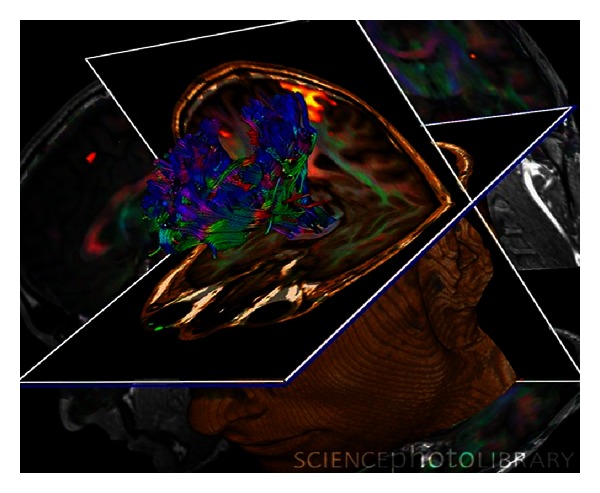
Brain tumour. Coloured 3D diffusion tensor imaging (DTI) and magnetic resonance imaging (MRI) scans of the brain of a 29-year-old with a low-grade glioma in the left frontal lobe. A DTI scan shows the bundles of white matter nerve fibers and is being used here for presurgical planning. The fibers transmit nerve signals between brain regions and between the brain and the spinal cord. A glioma arises from glial cells; nervous system supports cells. DTI scans show the diffusion of water along white matter fibers, allowing their orientations and the connections between brain regions to be mapped.
